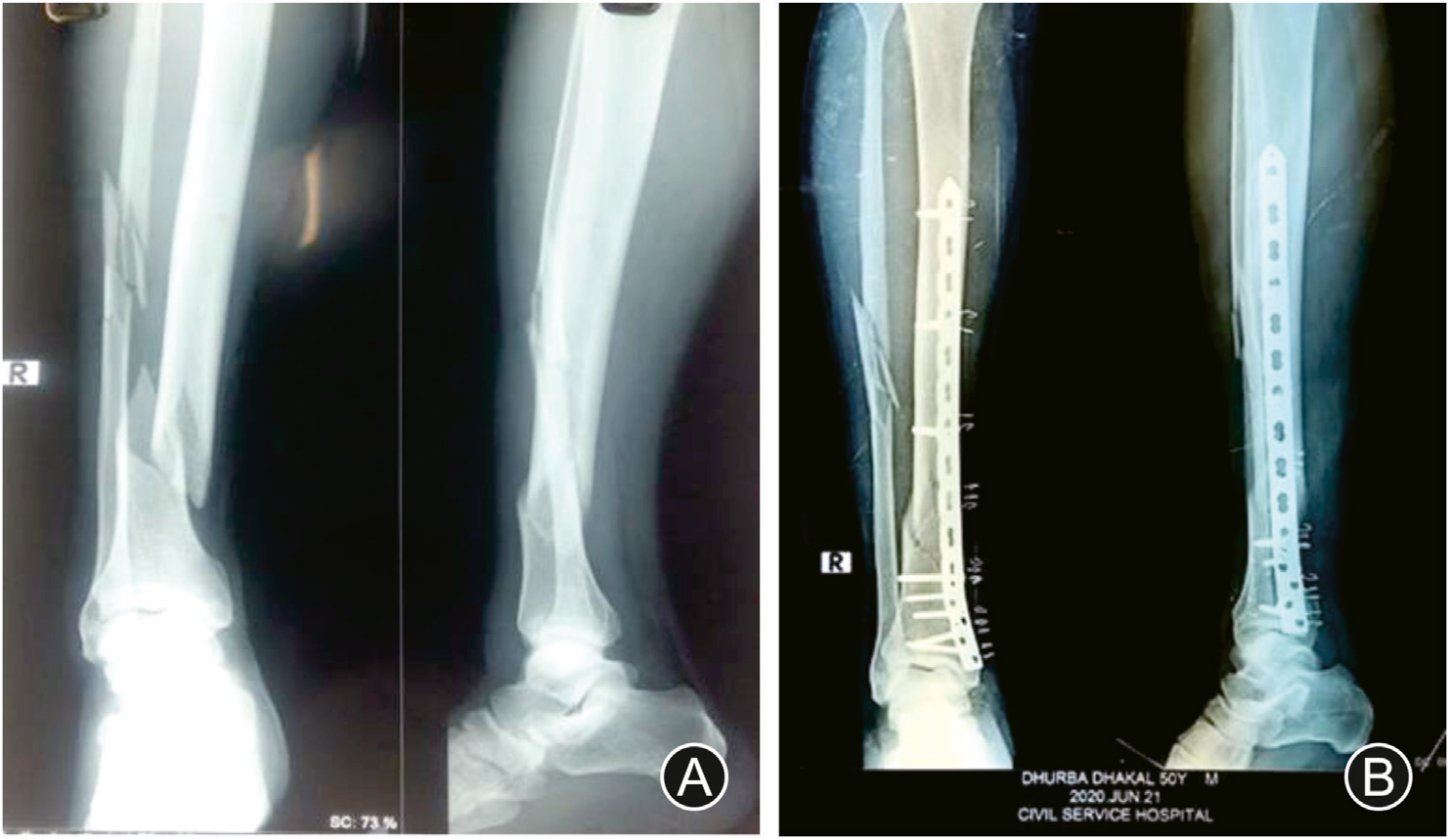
(A) Preoperative anteroposterior and lateral radiographs of distal third tibia and midshaft fibula fractures; (B) Immediate postoperative radiograph anteroposterior and lateral views fixed with precountered locking compression plate with MIPPO technique.
